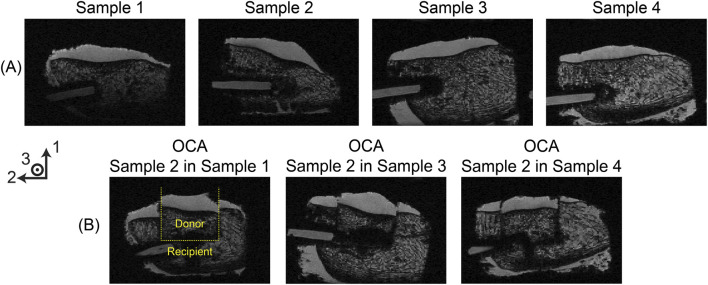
High-resolution MRI images in the 1-2 plane. (A) Intact patellae samples, (B) the same samples after the OCA transplantation. The first image shows the boundary between the donor graft and the recipient cartilage for reference.
